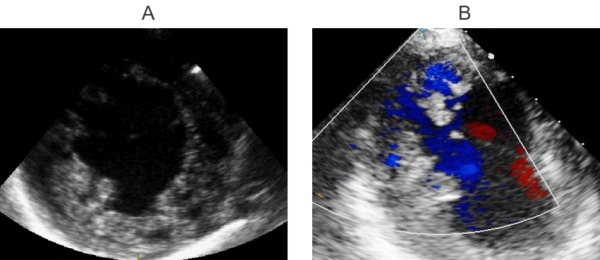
Transthoracic echocardiography showing left ventricular noncompaction of A) lateral wall at parasternal short axis view B) apical and apicolateral wall at apical 4-chamber view with noncompacted:compacted myocardium ratio of more than 2. Color doppler imaging revealed communication between myocardial recesses and left ventricular cavity.
